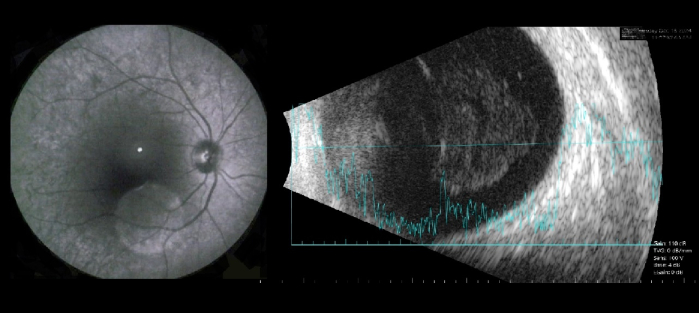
Left: The infrared image of the fundus reveals the central shadow of a detached vitreous body. Right: B-scan ultrasonography showing complete posterior vitreous detachment (PVD) with a shrunken vitreous body containing numerous fine echogenic opacities (gain: 110 dB).
